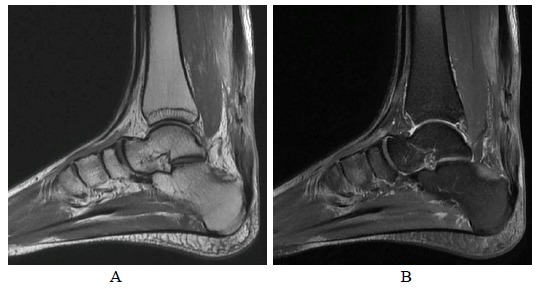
2 months after open surgery for Achilles repair in Patient A. PD-weighted and fat suppressed T2-weighted sagittal images show apparent tendon discontinuity at the operative site. Sutures are present at both ends with no significant separation.
